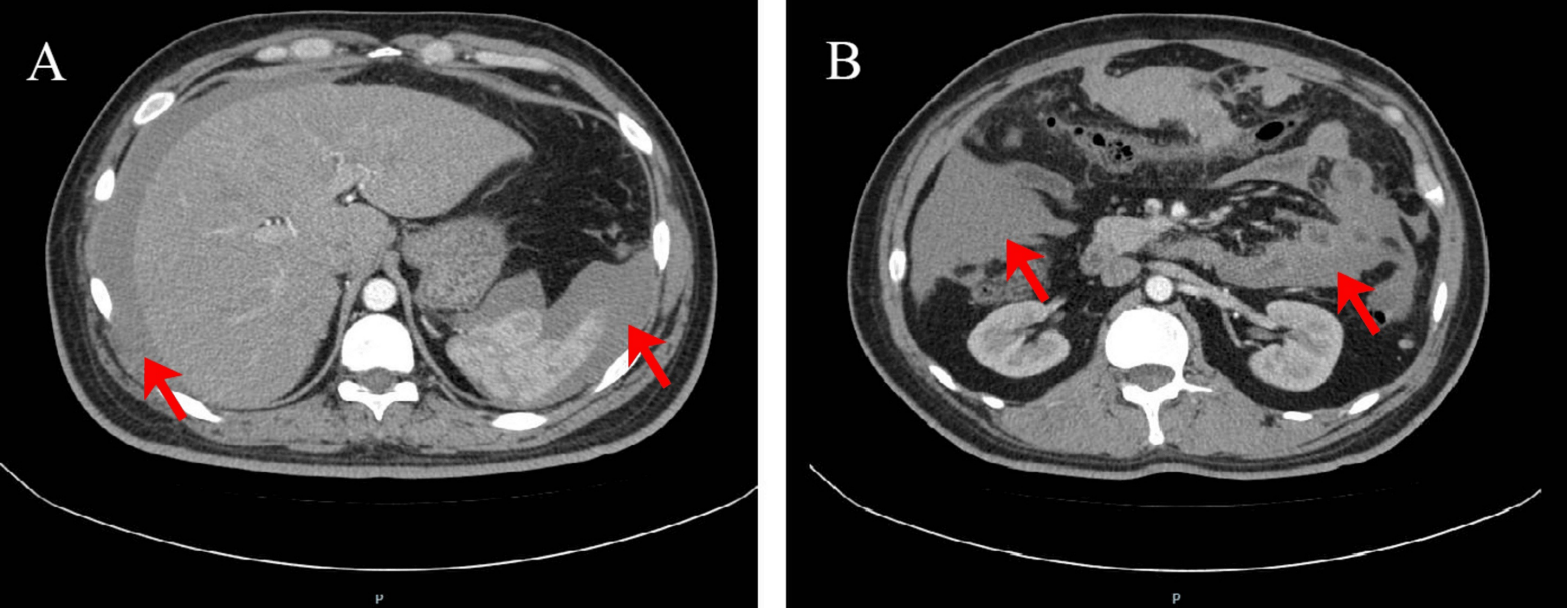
Contrast-enhanced abdominal CT Contrast-enhanced abdominal CT in axial planes, compared to the prior examination, reveals an increase in hemoperitoneum surrounding the liver and spleen (A, red arrow), along with an increased accumulation of blood within the interintestinal spaces (B, red arrow).
